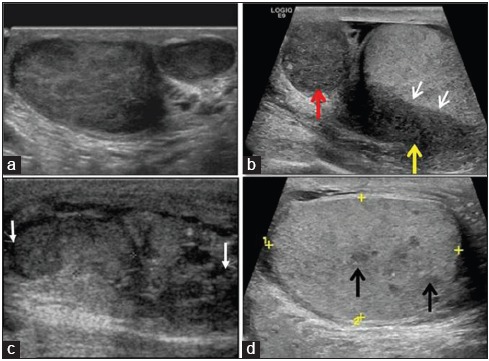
Various examples of gray scale sonographic patterns of testicular tuberculosis. (a) Diffusely enlarged right testis with heterogeneous hypoechoic pattern, (b) diffusely enlarged head (red arrow) and body (yellow arrow) of epididymis with infiltration of adjacent testes parenchyma (white arrows) showing homogenous hypoechoic pattern, (c) nodular enlarged heterogeneously hypoechoic testes, with ill-defined nodules (small white arrows) virtually indistinguishable from tumor, (d) ill-defined tiny hypoechoic nodules (black arrows) in testicular parenchyma.
